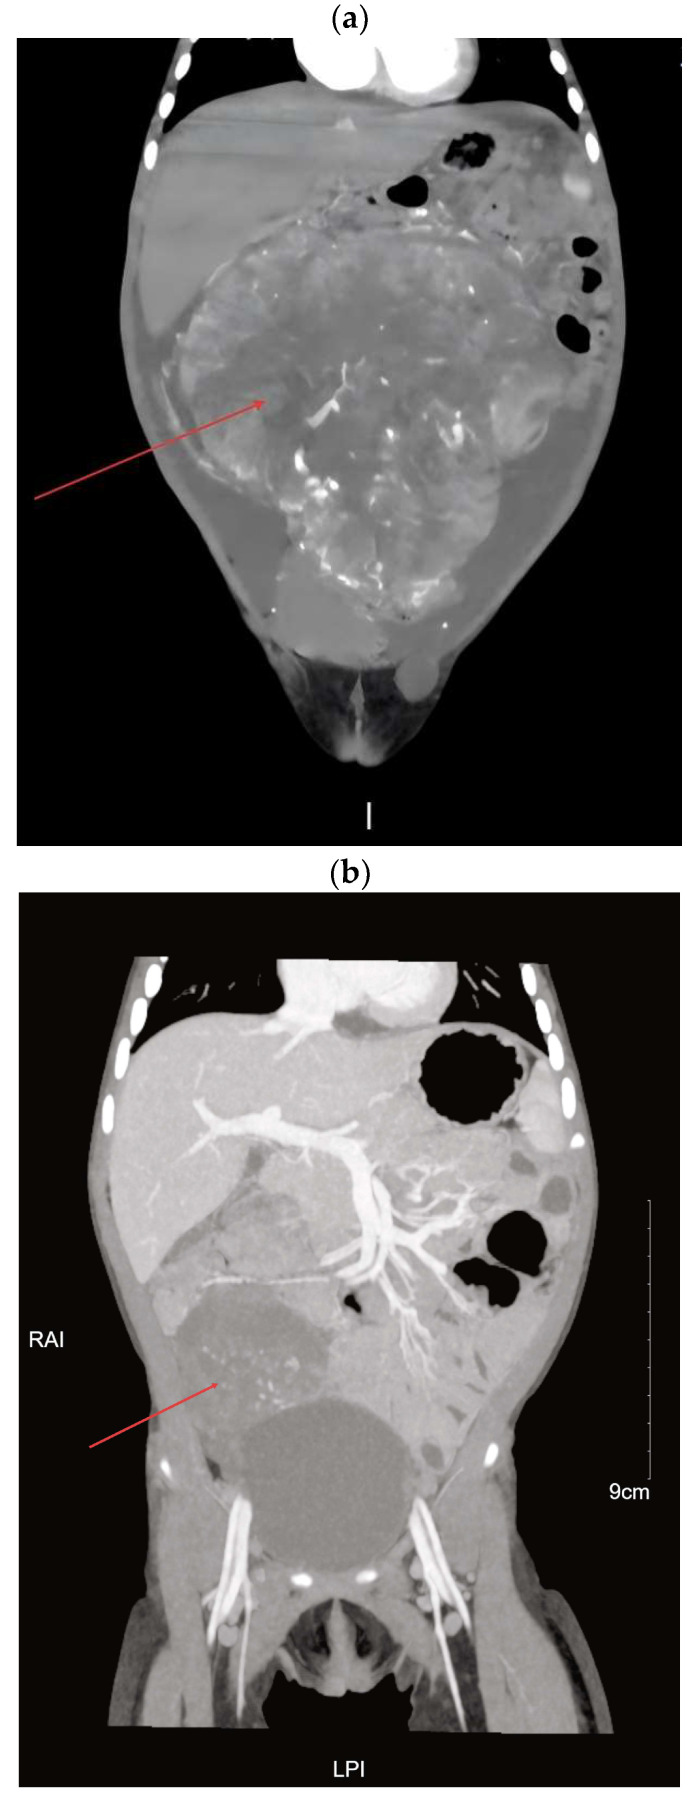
Enhanced abdomen CT demonstrates changes in tumor size before and after chemotherapy, and the arrow indicates the tumor before and after chemotherapy: (a) tumor before chemotherapy; (b) tumor after two cycles of chemotherapy.
